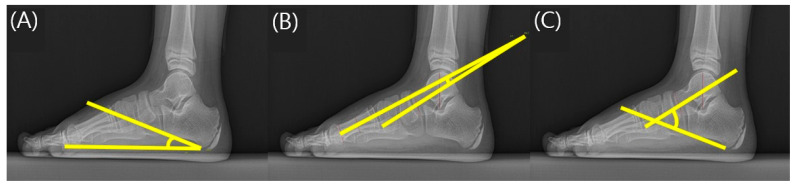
Measurement of radiographic parameters. (A) Calcaneal pitch angle; (B) Talometatarsal angle; (C) Talocalcaneal angle.
